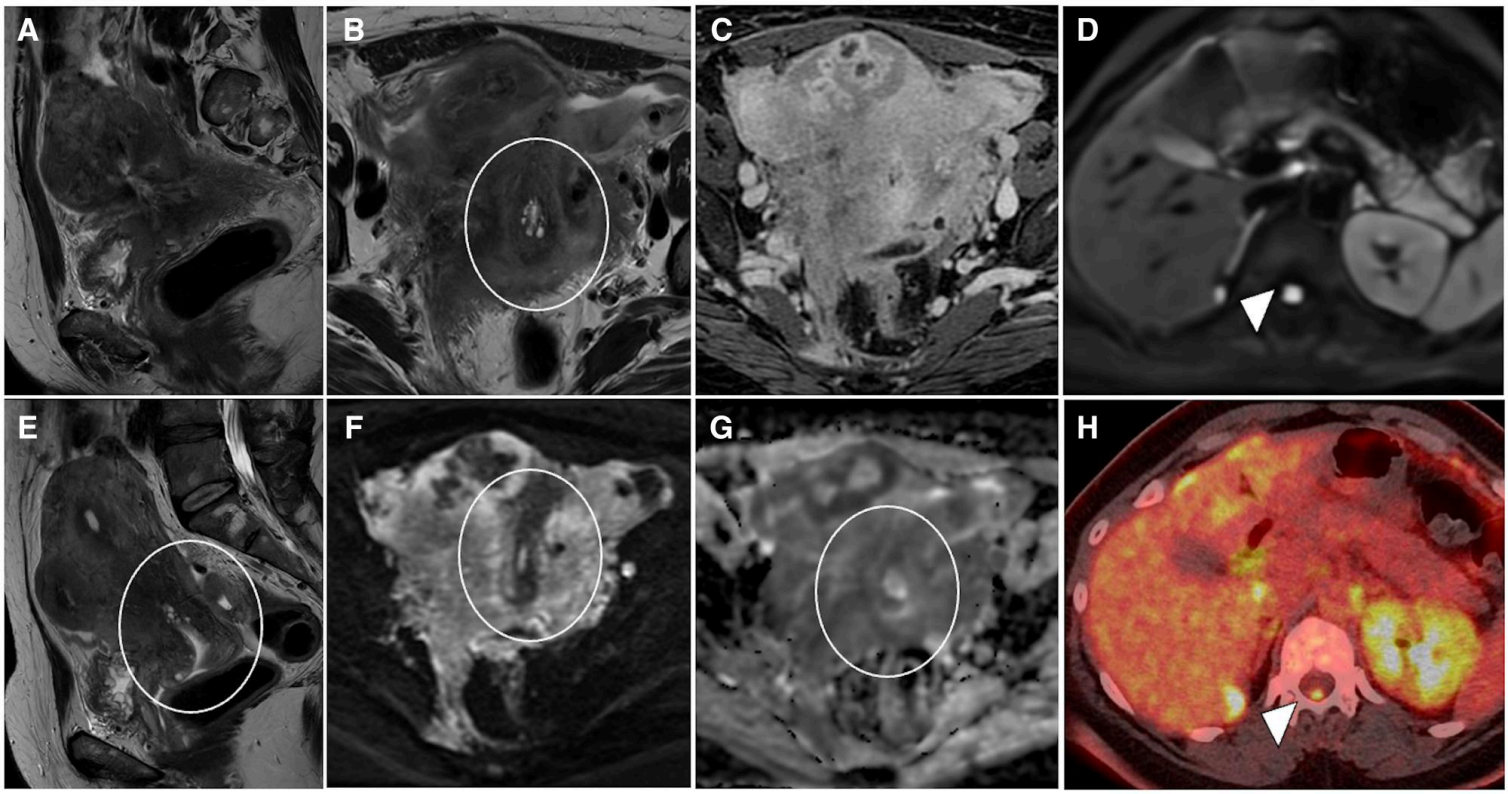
Diffuse large B-cell cervical lymphoma. On MRI, symmetrical and diffuse uterus enlargement can be noted due to lymphomatous infiltration. Despite the lesion size, no area of haemorrhage or necrosis is visible on postcontrast imaging (C). Preservation of the cervical mucosal layer (white circle), which is a distinguishing feature, is seen on axial and sagittal T2-WI (B, E), DWI images (F) and ADC map (G). Careful evaluation of additional disease sites (arrowheads) is needed, as seen on upper abdomen DWI (D) and FDG-PET/CT images (H). Abbreviation: WI = weighted imaging.
